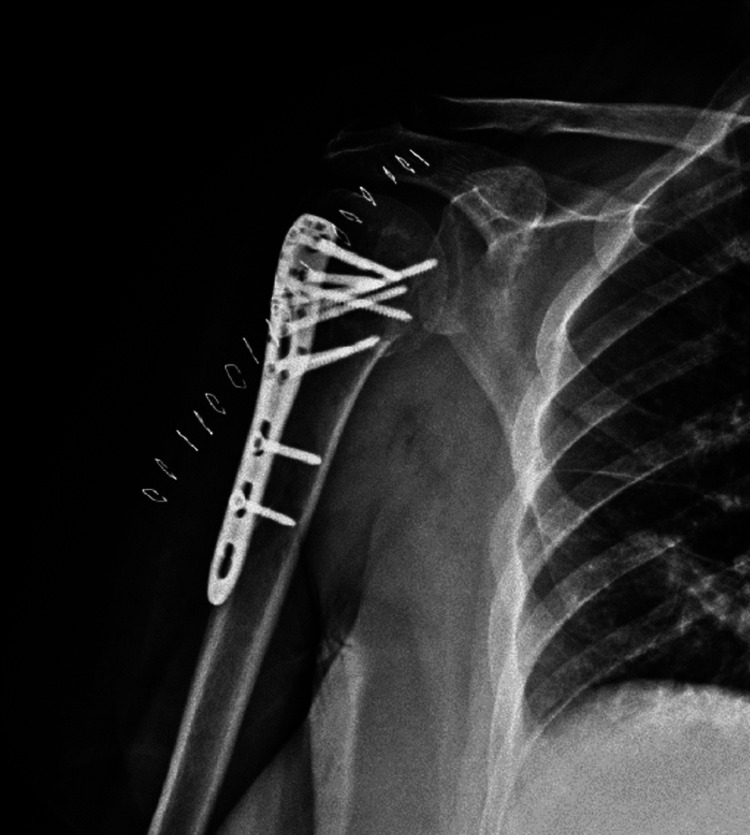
Locking plate osteosynthesis postoperative X-ray.
